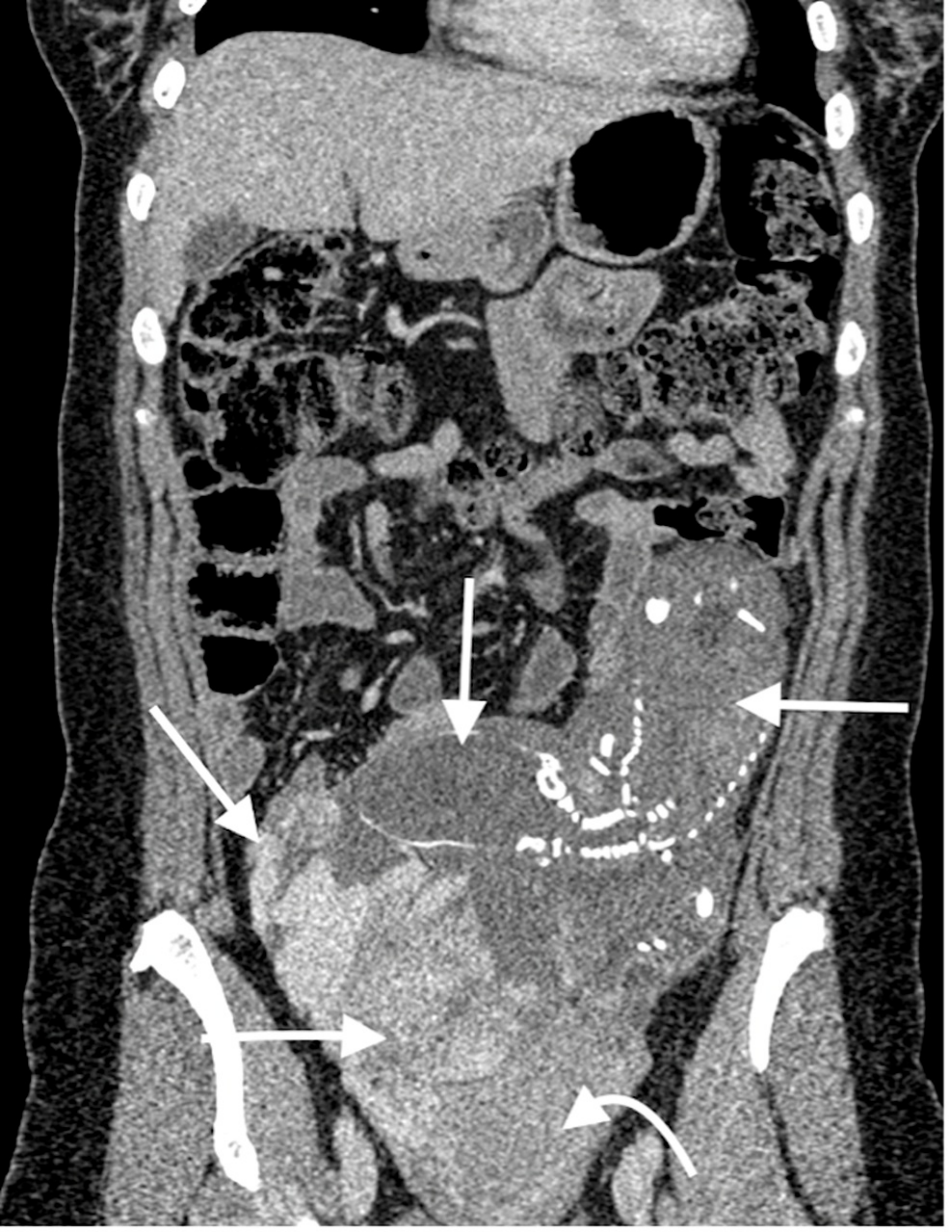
Coronal, post-contrast CT image showing scaphocephaly and hydrocephalus of the fetal head (vertical arrow), the fetal body located on the left side of peritoneal cavity (horizontal arrow pointing to right), uterine body (curved arrow), hypervascular placental tissue (horizontal arrow pointing to left), and early filling of the right ovarian vein (oblique arrow).
